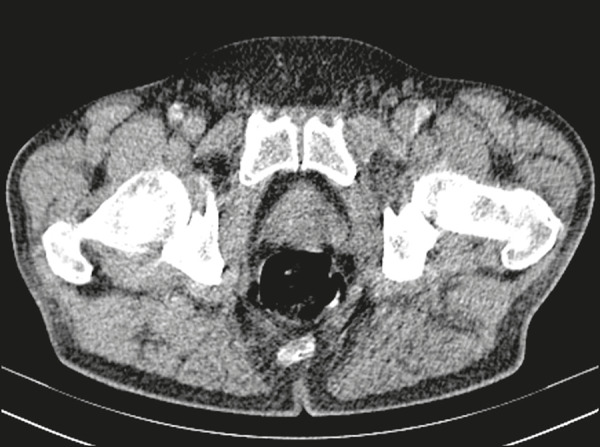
Computed tomography performed four weeks after the treatment, with no residual or new prostatic collections.
